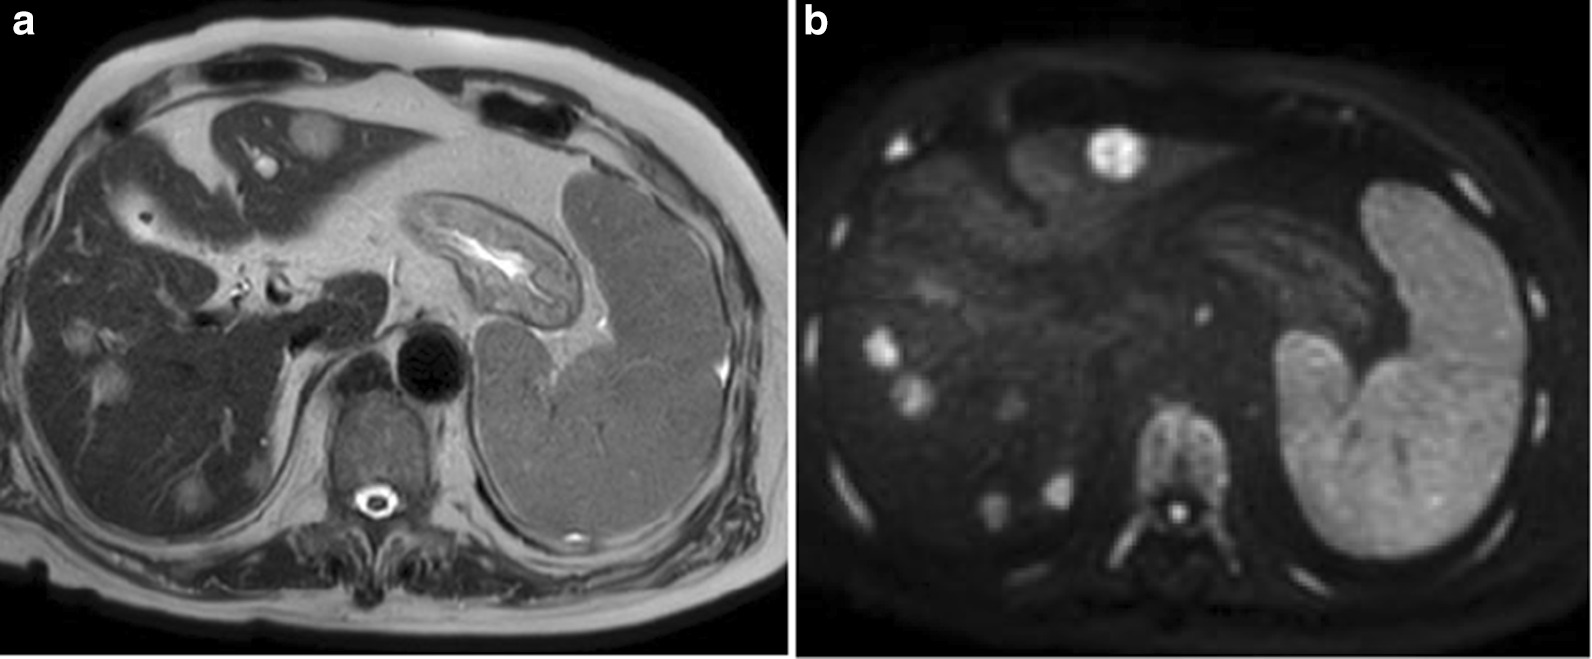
Magnetic resonance imaging findings. a Hepatic lesions were hyperintense on T2-weighted images, b marked signal restricted on diffusion-weighted images. Splenomegaly without focal lesions was also seen
